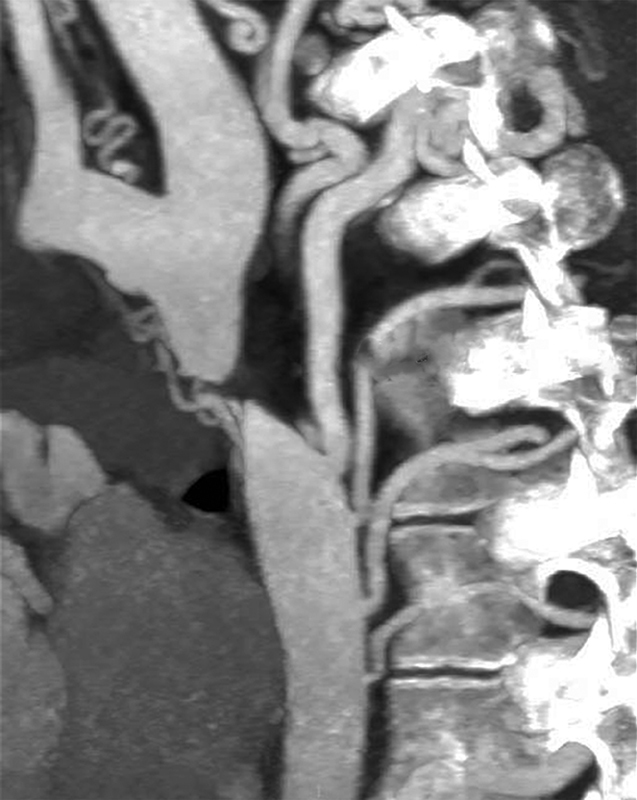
Preoperative computed tomography scan showing complete aortic arch interruption below the left subclavian artery with extensive arterial collateralization.
